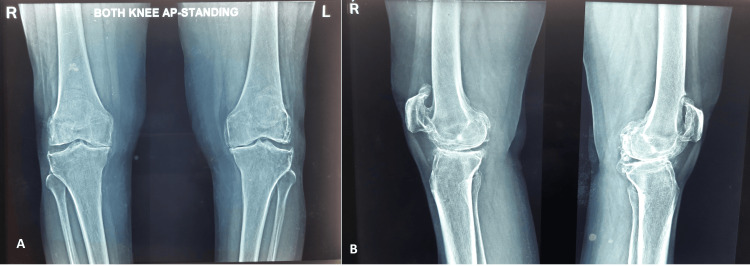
(A and B) Preoperative radiographs of the diseased knee.
